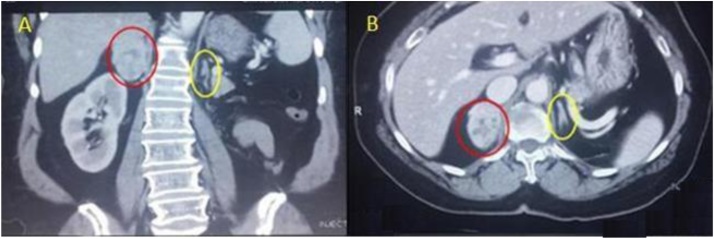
contrast enhanced CT scan of the abdomen. Coronal (A) and axial (B) sections showing an enhanced right adrenal mass (red circle) and normal looking left adrenal gland (yellow circle).
